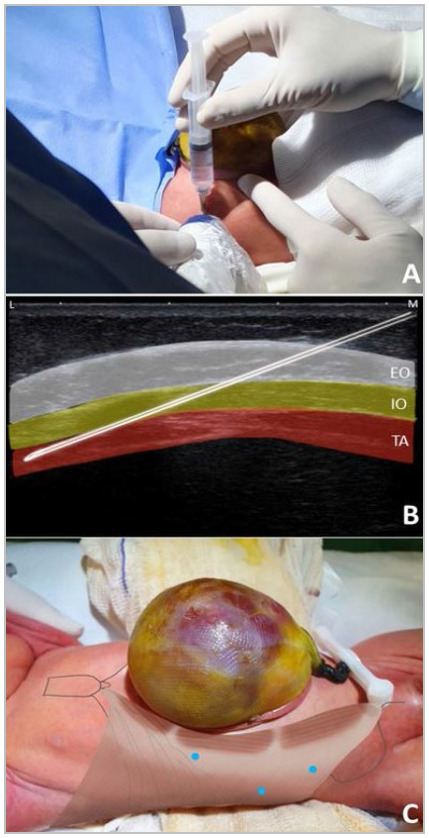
BTA application technique on the lateral abdominal wall of a newborn with GO. (A) The transducer is placed transversely and the needle in the medial-lateral direction. (B) Ultrasound image with the three differentiated muscle layers and correct positioning of the needle; white: external oblique muscle, yellow: internal oblique muscle, red: transversus abdominis muscle. (C) Blue dots representing injection sites.
